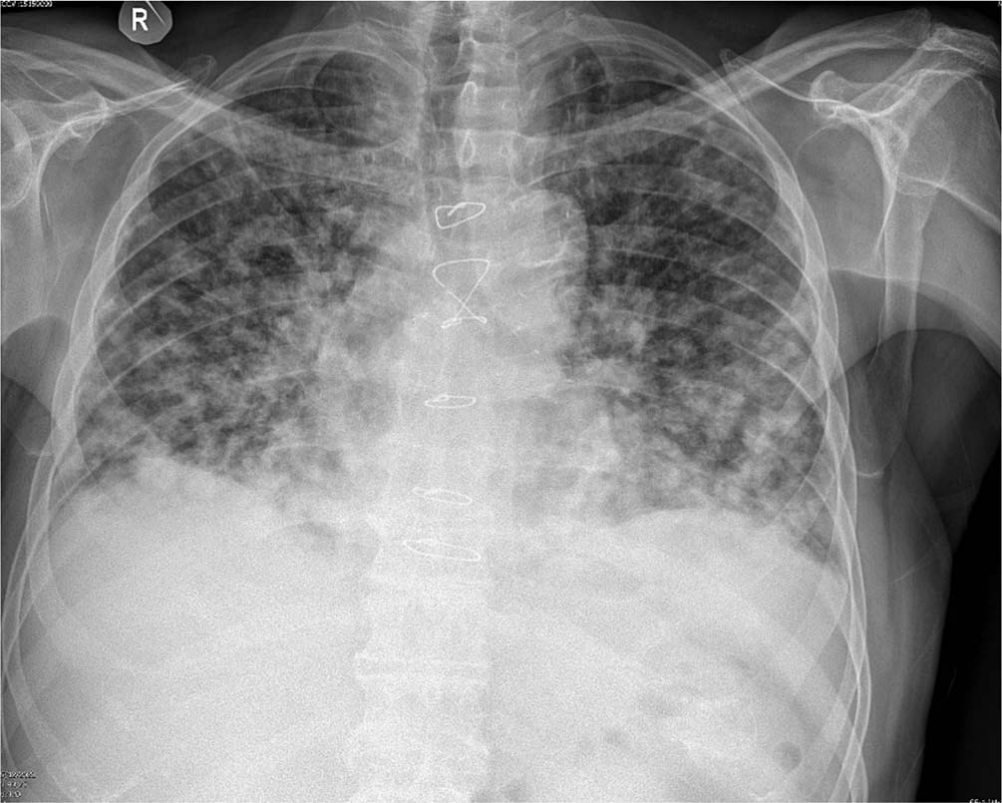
Chest X-ray eight months after TURP.
